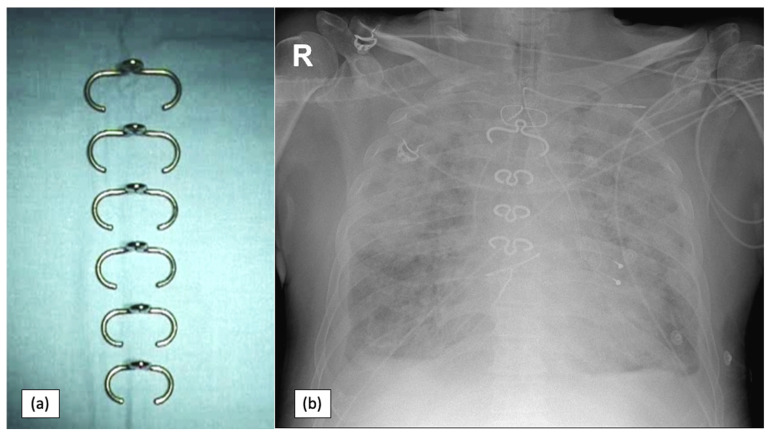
Nitinol clips: (a) different sizes used for sternal closure; (b) chest X-ray: aspect of sternal closure using nitinol clips, R—right side.
